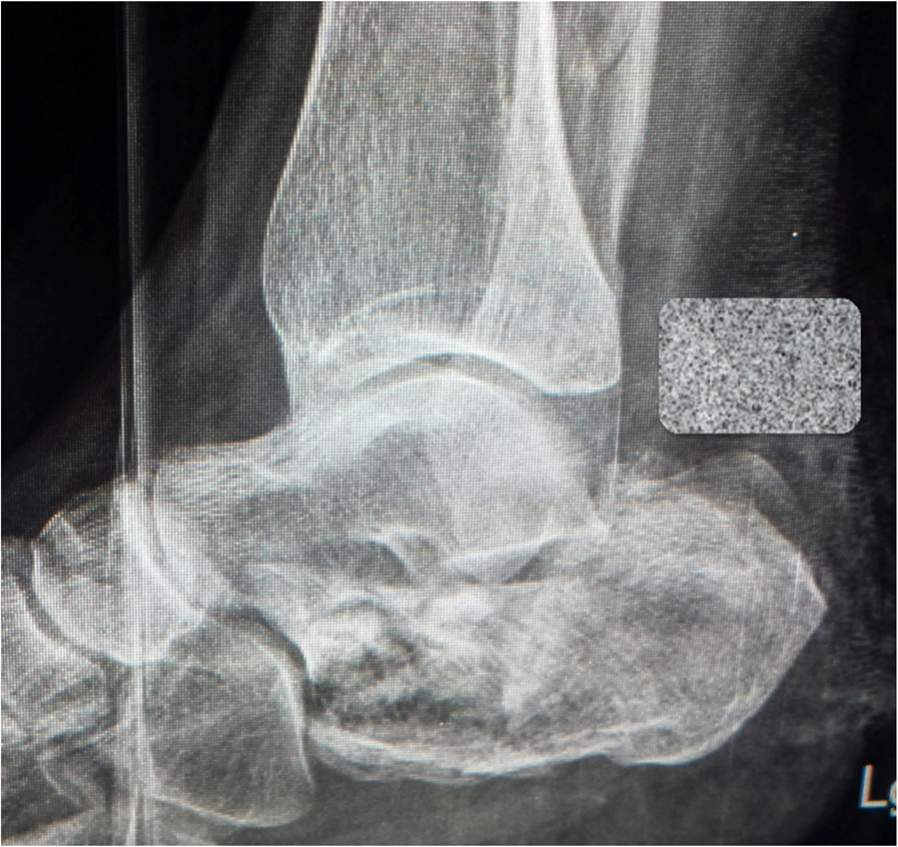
X-ray of calcaneal fracture after injury
